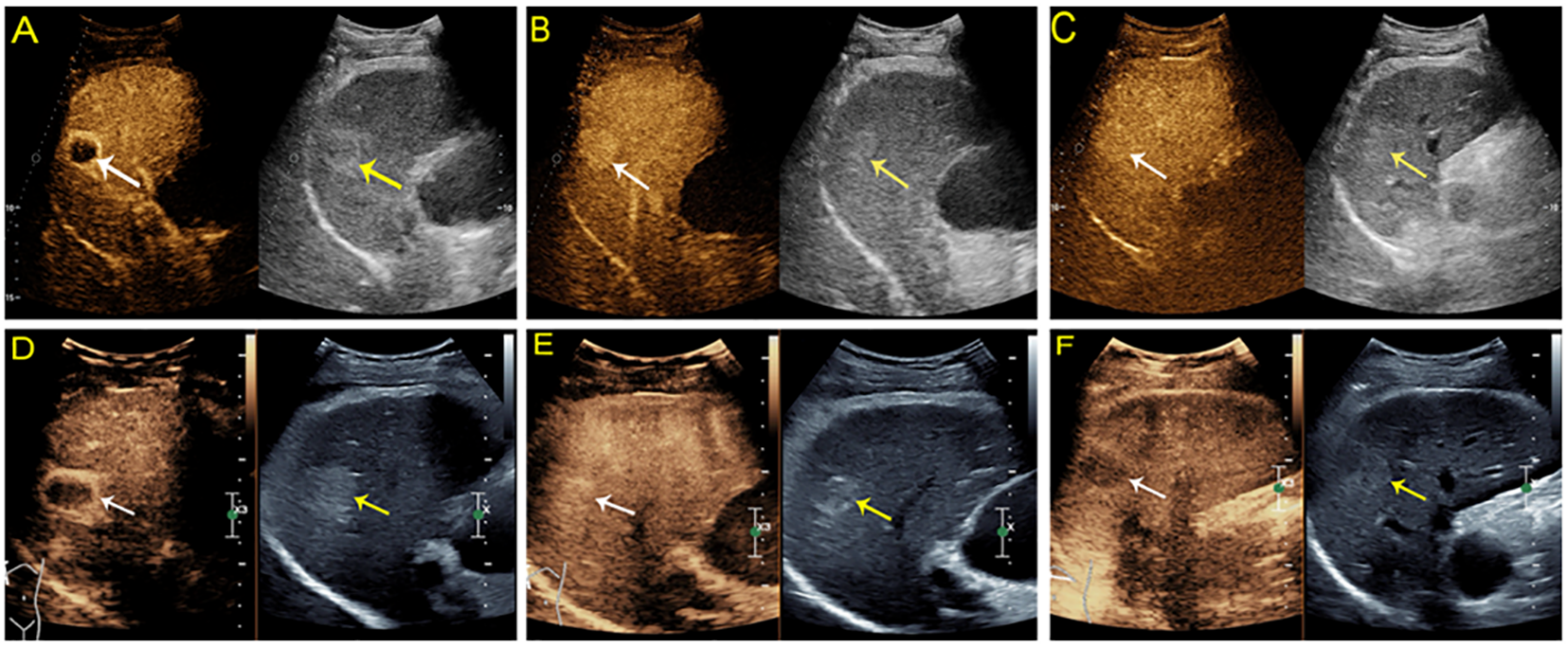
Contrast-enhanced US images from a 51-year-old man with chronic hepatitis B. A hyperechoic solid lesion measuring 2.9 cm in segment VI of the liver was detected on baseline US. On SonoVue enhanced-US, the nodule showed rim arterial phase hyperenhancement (APHE) (A, white arrow) followed by homogeneous hyperenhancement in two minutes and thirty seconds (B, white arrow) and iso- to mild hyperenhancement in the late phase (C, white arrow). On Sonazoid-enhanced US, the nodule appeared to have rim APHE (D, white arrow) and iso- to mild hyperenhancement in two minutes (E, white arrow), followed by hypoenhancement in ten minutes and nineteen seconds in the Kupffer phase (F, white arrow). The nodule was confirmed to be a hemangioma by contrast-enhanced MRI seven months later.
